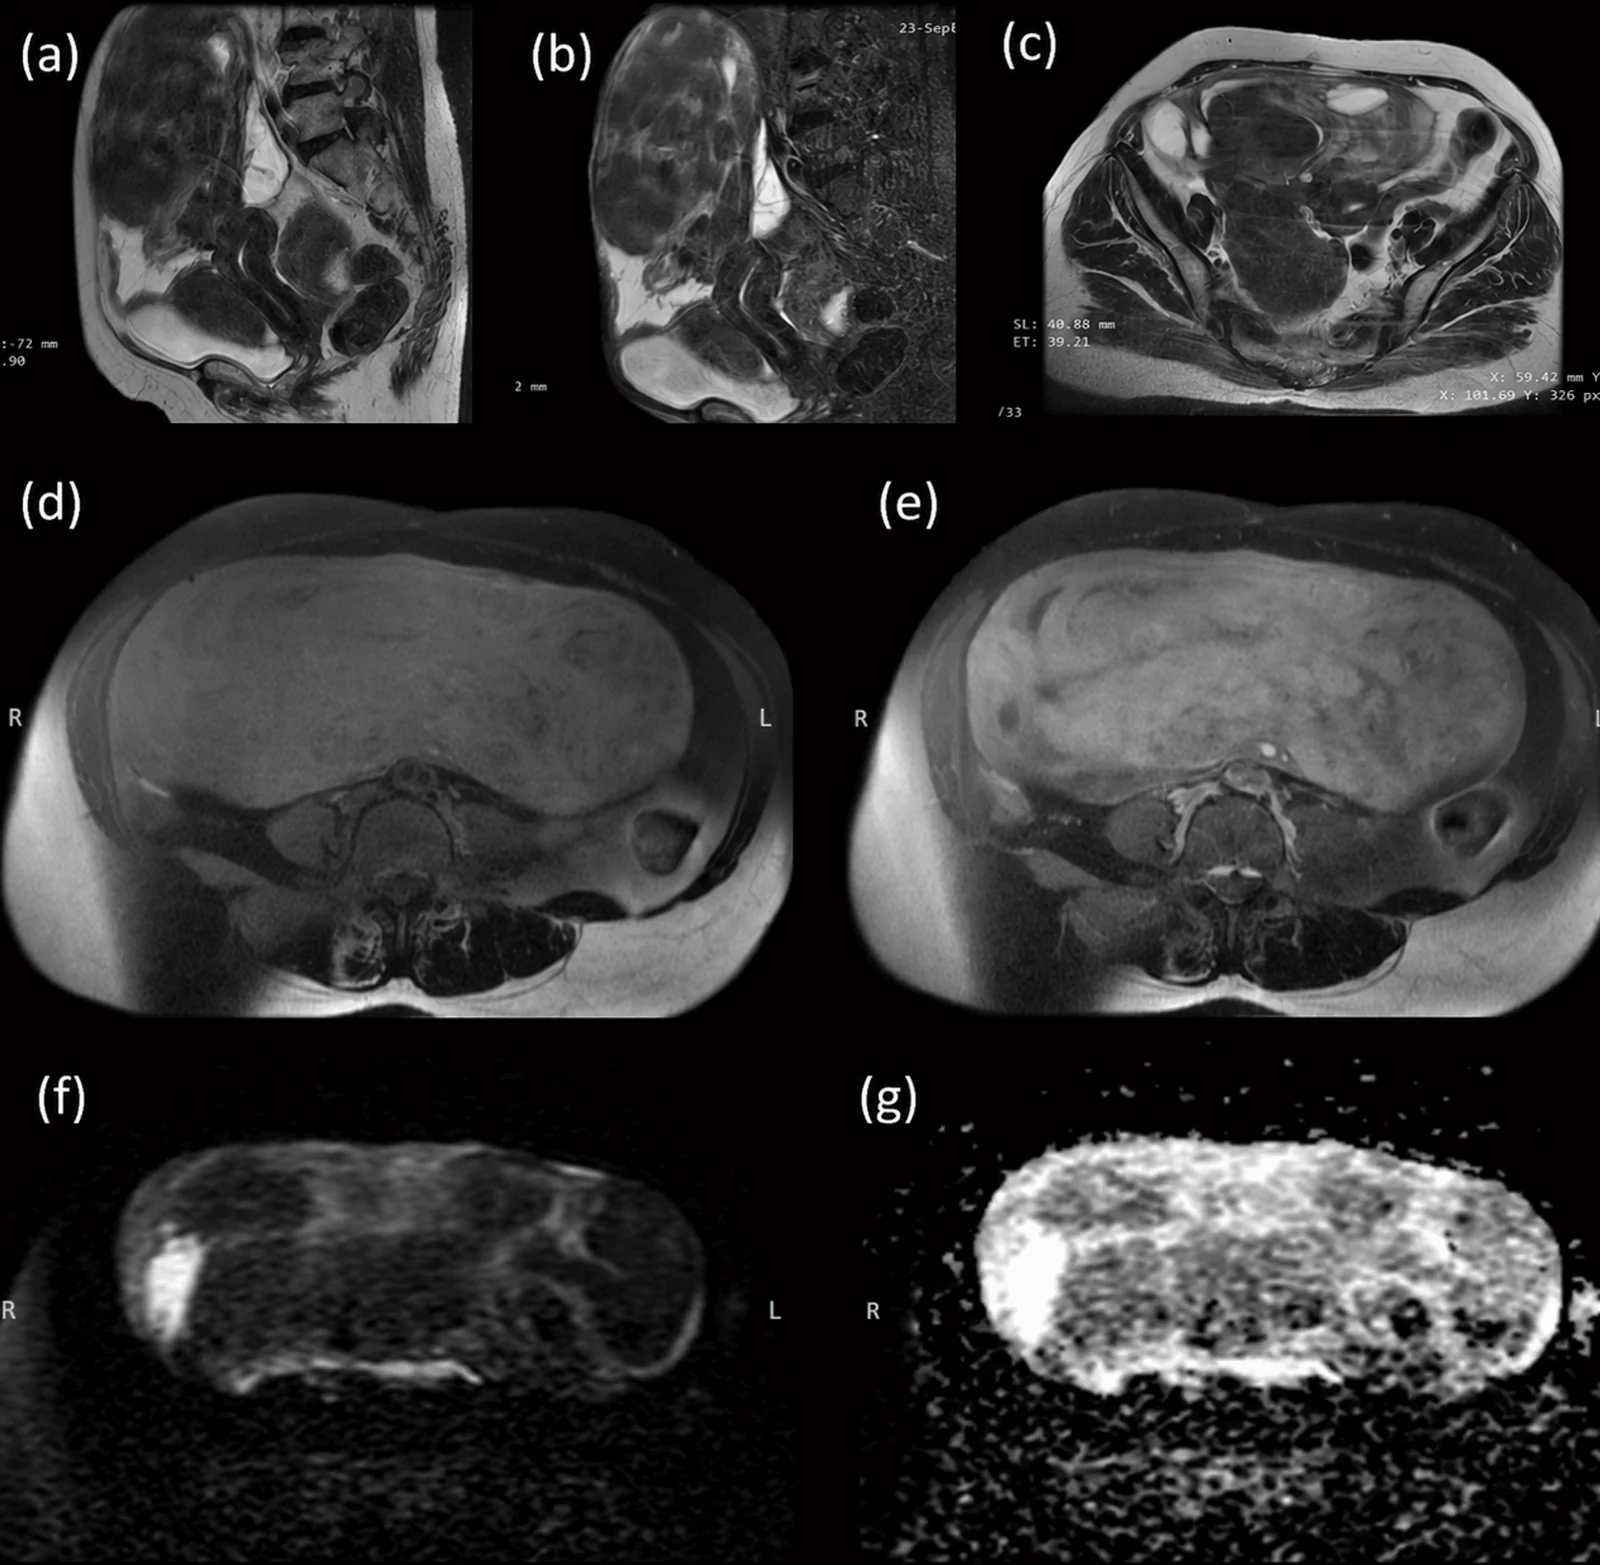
Magnetic resonance imaging findings (a,b) Sagittal T2-weighted images demonstrate a large, well-circumscribed, multilobulated, predominantly solid pelvic mass with areas of cystic degeneration. (c) Axial T2-weighted image showing the mass occupying the pelvis and displacing adjacent pelvic structures. (d) Axial T1-weighted image showing predominantly isointense signal within the solid component. (e) Post-contrast T1-weighted image demonstrating mild gradual enhancement of the solid component. (f) Diffusion-weighted image and (g) corresponding apparent diffusion coefficient map showing no diffusion restriction.
